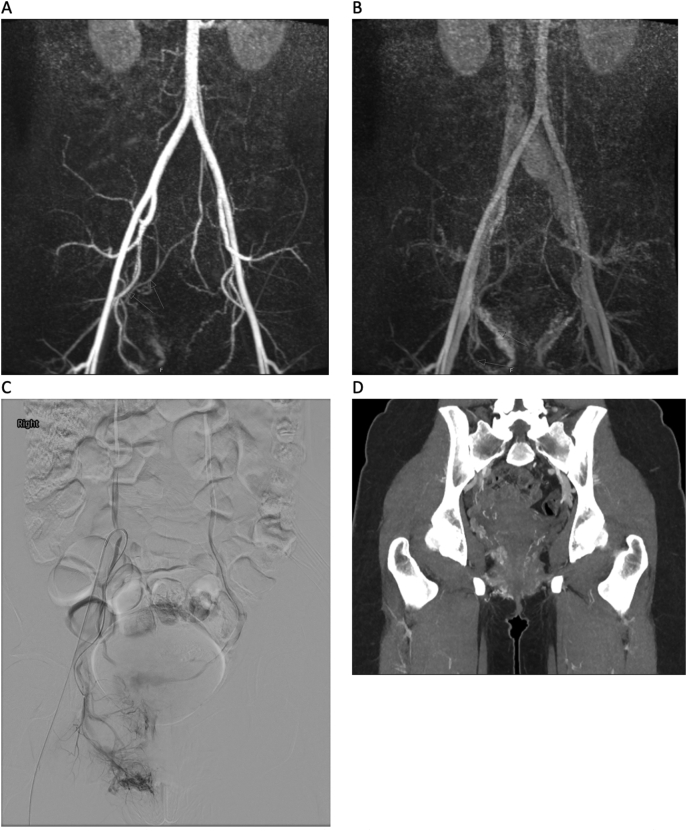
A: Coronal slice of her MRI pelvis demonstrating feeders from her right internal iliac artery as well as the inferior mesenteric artery and superior rectal artery. B: Draining veins were seen to predominantly course to the right internal iliac vein. C: DSA performed via right internal iliac artery with right sided haemorrhoids seen secondary to venous hypertension from outflow obstruction. D: Post embolisation CTMA demonstrating a diminished rectal component.
